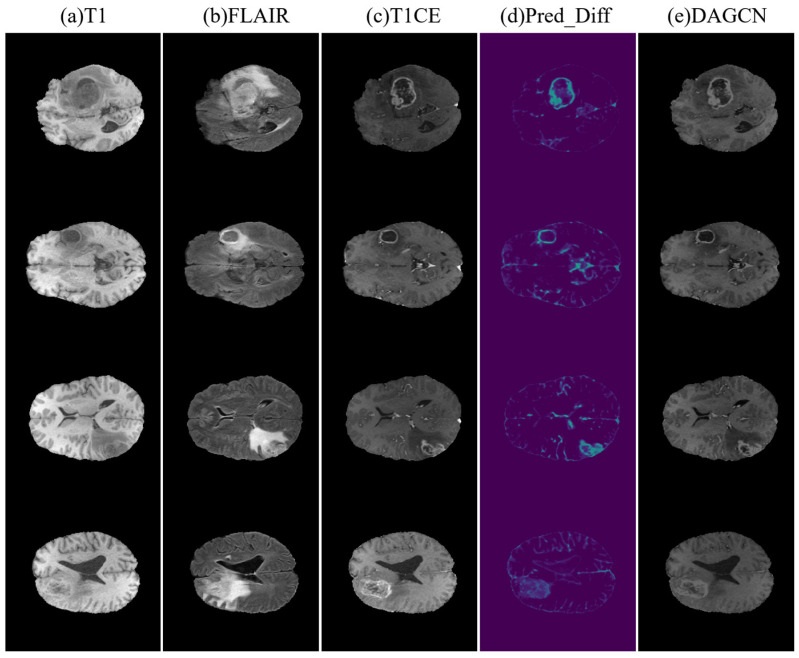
Representative generation process of DAGCN under FLAIR-guided synthesis. For each case, the non-contrast T1-weighted images, auxiliary FLAIR image, predicted discrepancy map, synthesized T1-CE image, and ground-truth T1-CE image are shown. Compared with T2 guidance, FLAIR guidance yields more spatially focused discrepancy maps, cleaner background suppression, and better delineation of enhancing lesion boundaries. Predicted discrepancy maps are displayed using the perceptually uniform viridis sequential colormap with a consistent scale, following recommendations for scientific color use [42].
